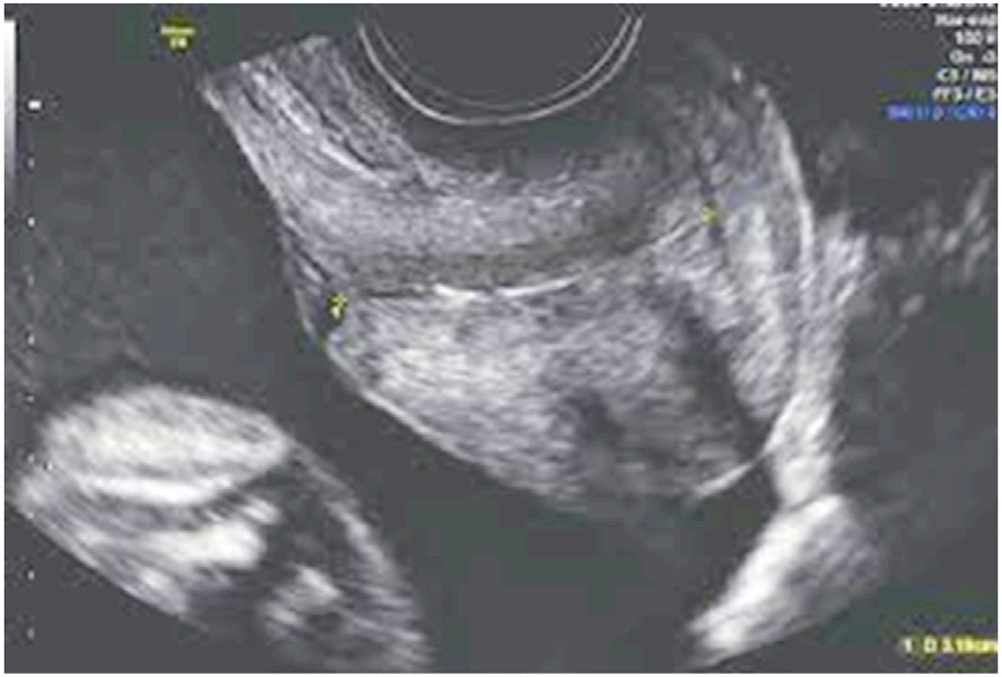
Determination of cervical length by ultrasound
